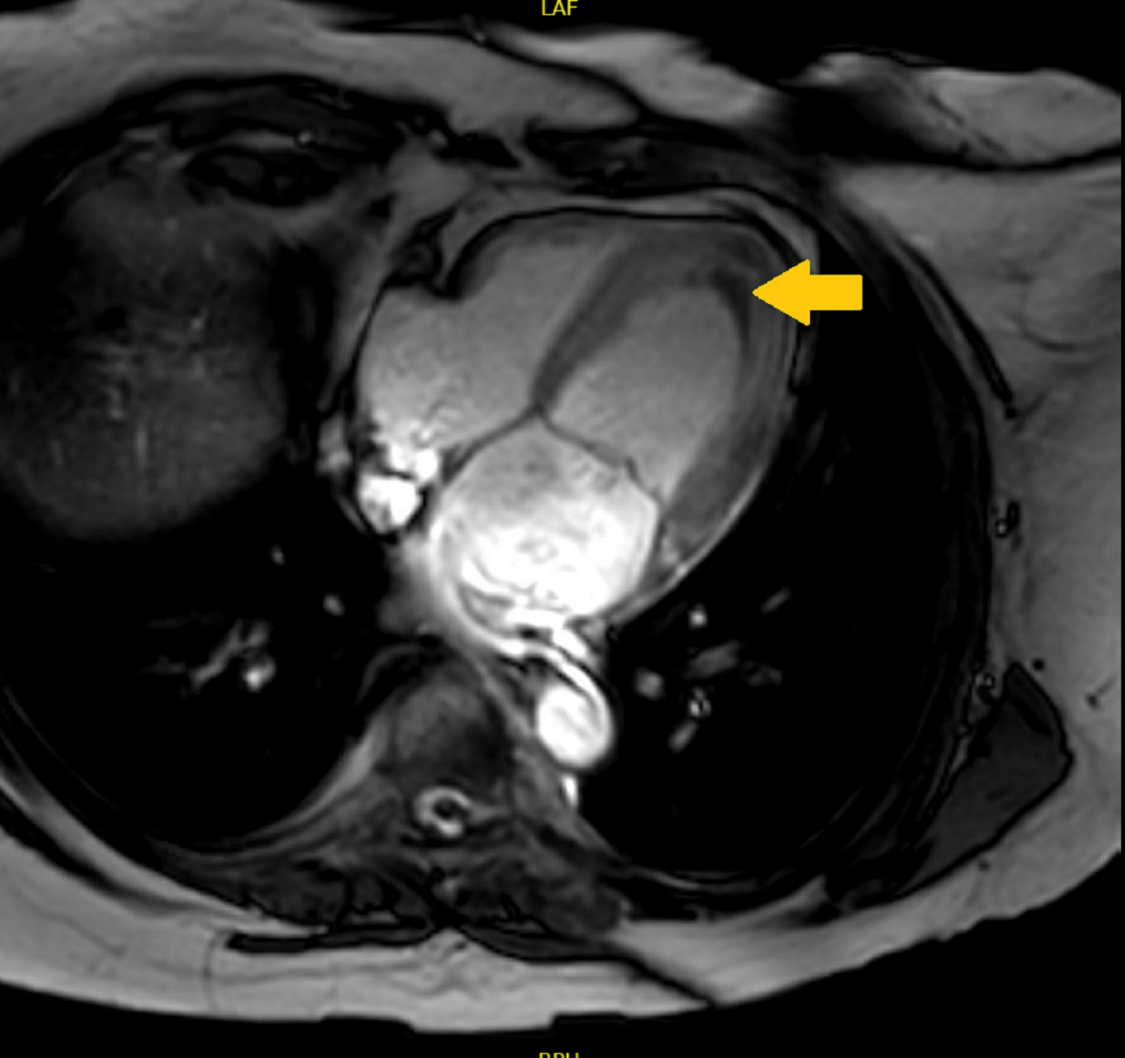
Cardiac MRI Cardiac MRI axial view showing well-defined area of low signal intensity consistent with thrombus formation (arrow)
